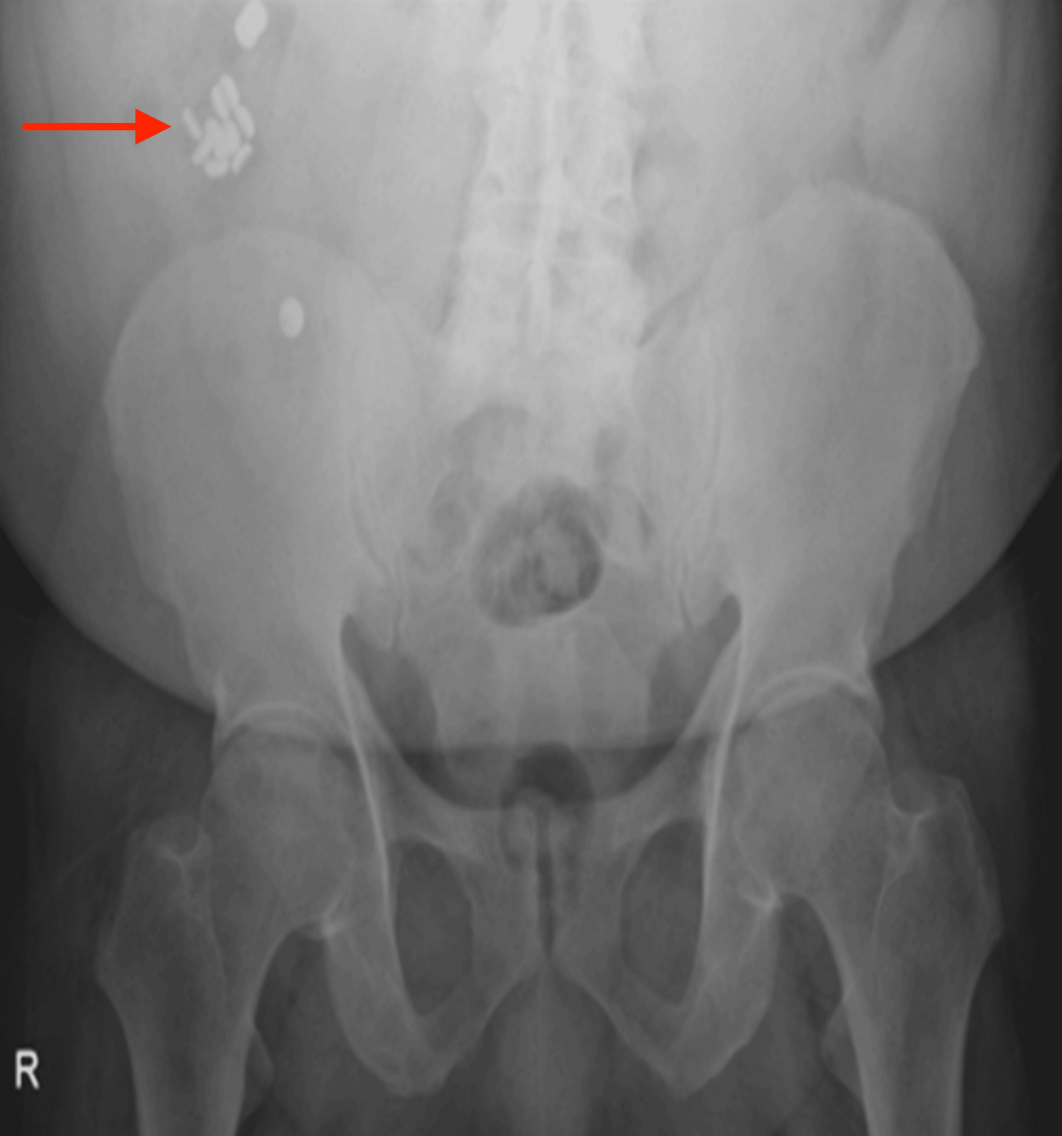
Initial upright abdominal X-ray showing multiple radiopaque foreign bodies seen mainly in the right lower quadrants (red arrow).
